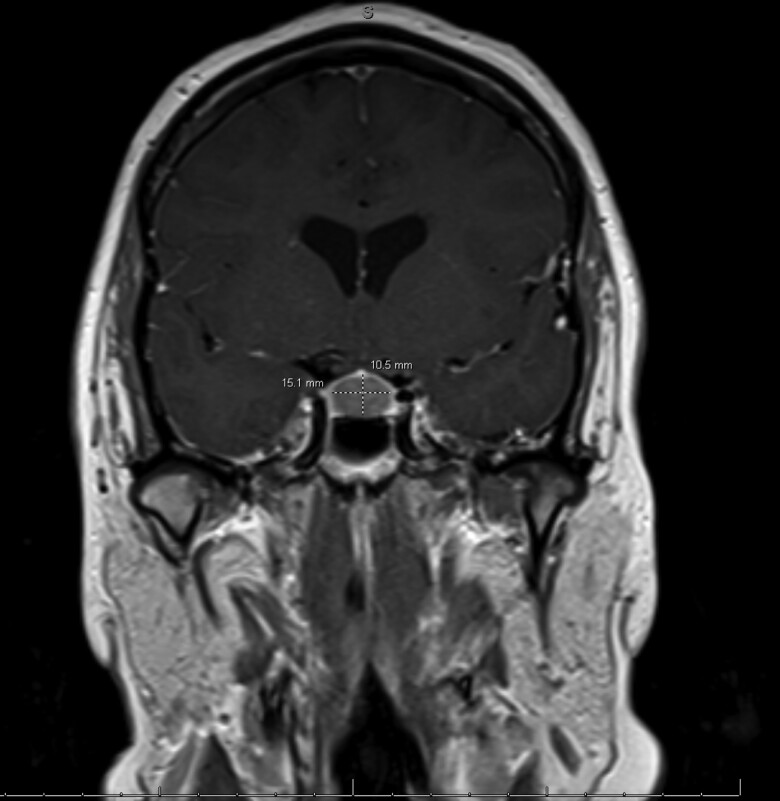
Magnetic resonance imaging brain with without contrast; T1 coronal image showing diffuse enlargement of the pituitary gland: 15.1 mm transverse × 10.5 mm craniocaudad.
